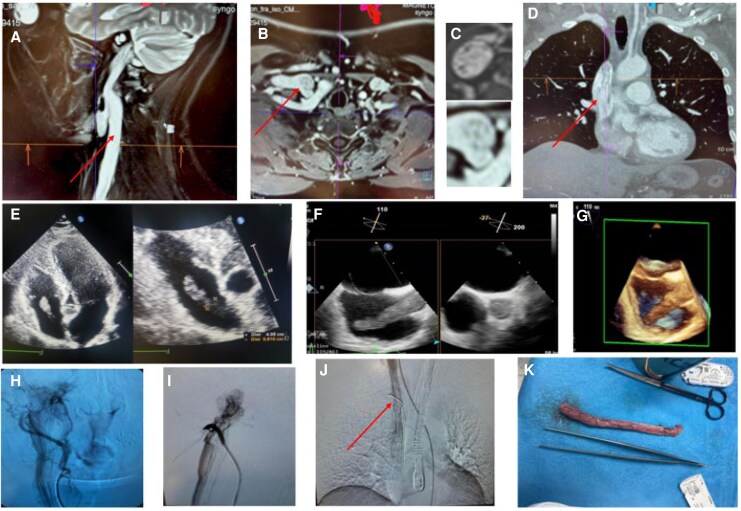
(*A*) MRI of the head and neck, the tumour spreading from the jugulotympanic region down via venous vascular system (arrow). (*B*) Axial MRI of the venous angle, where the internal jugular vein joins the subclavian vein, showing a tumour with a characteristic ‘salt-and-pepper’ appearance (arrow). (*C*) Magnified MRI view showing the ‘salt-and-pepper’ sign, reflecting flow voids (‘pepper’) and hyperintense tumour tissue (‘salt’), typically seen in highly vascular tumours. (*D*) Chest CT showing the tumour extending from superior vena cava into the right atrium (arrow). (*E*) TTE, apical view, showing a tumour in the right atrium reaching the level of the tricuspid valve. Echocardiographic assessment showed a normal right heart with no TR, no RA dilatation and no pulmonary hypertension. The endocardial portion of the mass measures approximately 4 cm in length and almost 1 cm in diameter. (*F*) TEE 2D: the tumour protruding from superior vena cava to the right atrium in bicaval view (on the left); superior vena cava in transverse section completely occupied by the tumour (on the right). (*G*) TEE: A finger-like tumour on 3D imaging. (*H*) Non-selective angiography of the right external carotid artery showing pathological tumour vascularization. (*I*) Selective angiography of the right occipital artery arising from the right external carotid artery showing pathological tumour vascularization. (*J*) TEE-guided biopsy performed during the angiography procedure. The mass was captured with a vascular loop, and a fragment was aspirated using the 12F Penumbra Indigo Lightning system (biopsy loop indicated by an arrow). Both angiography and embolization procedures were performed via transradial access (TRA, 7F). (*K*) Macroscopic view of the resected tumour. A venotomy of the internal jugular vein was performed. The tumour, hanging loosely, was extracted from the superior vena cava and right atrium. The upper portion of the mass was mobilized with gentle traction and then resected using electrocautery. The excised tumour measured approximately 18 cm in length.
